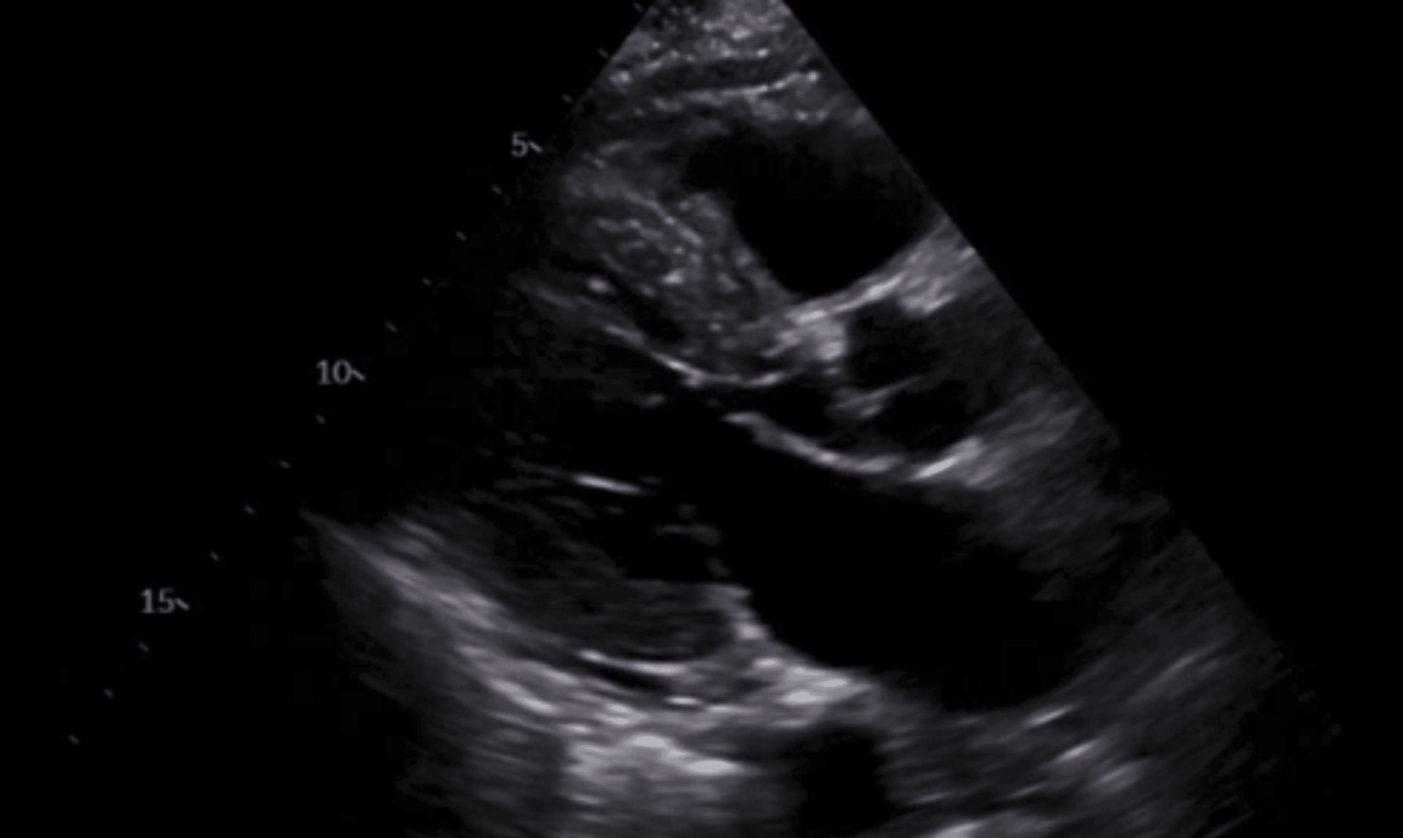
Trans-TTE showing a preserved EF of the left ventricle TTE, thoracic echocardiogram; EF, ejection fraction
